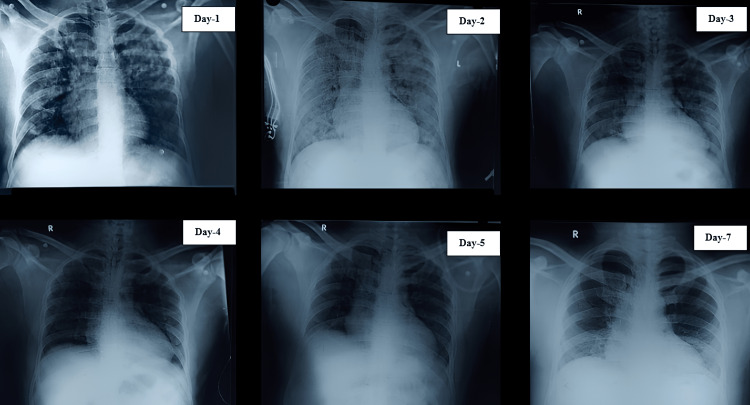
Chest X-ray findings on various days. Bilateral opacities in all lung lobes from Day 1 to Day 4 and is diagnosed as pneumonitis.
